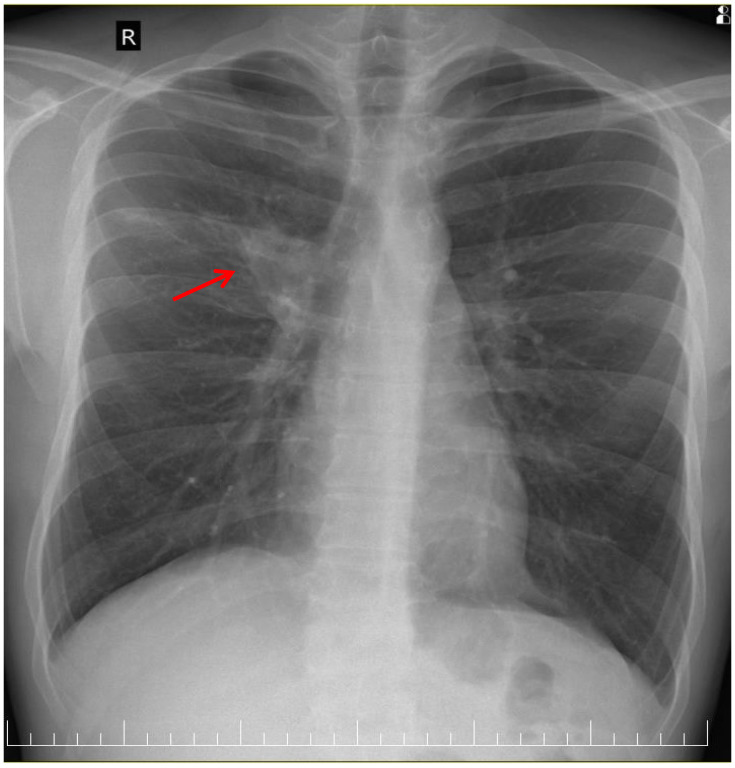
Chest X-ray showing high-intensity opacity in right suprahilar region (red arrow).
